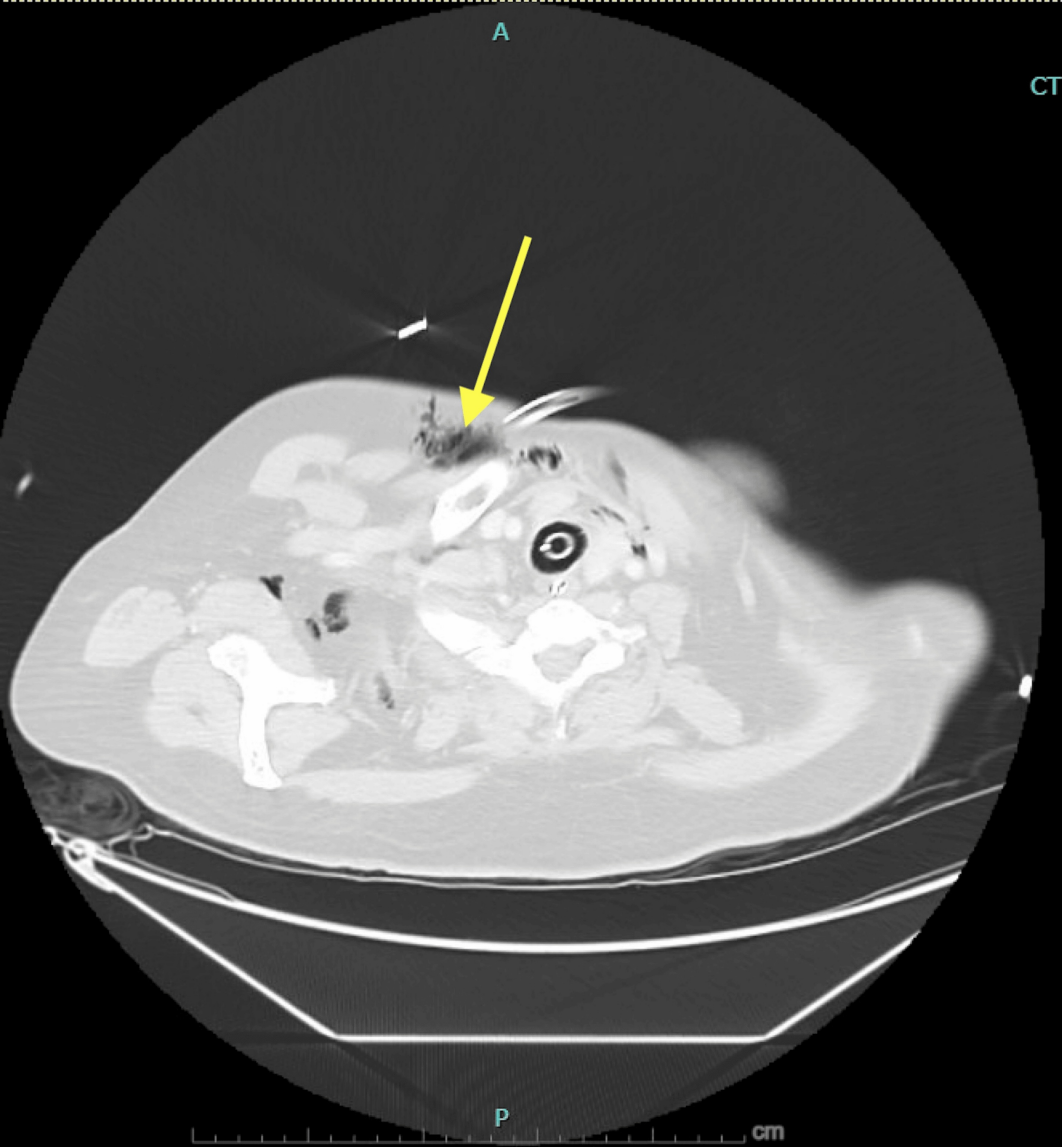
CT of the chest demonstrating subcutaneous emphysema. The image demonstrates subcutaneous emphysema in the anterior chest wall (yellow arrow). CT: computed tomography.
